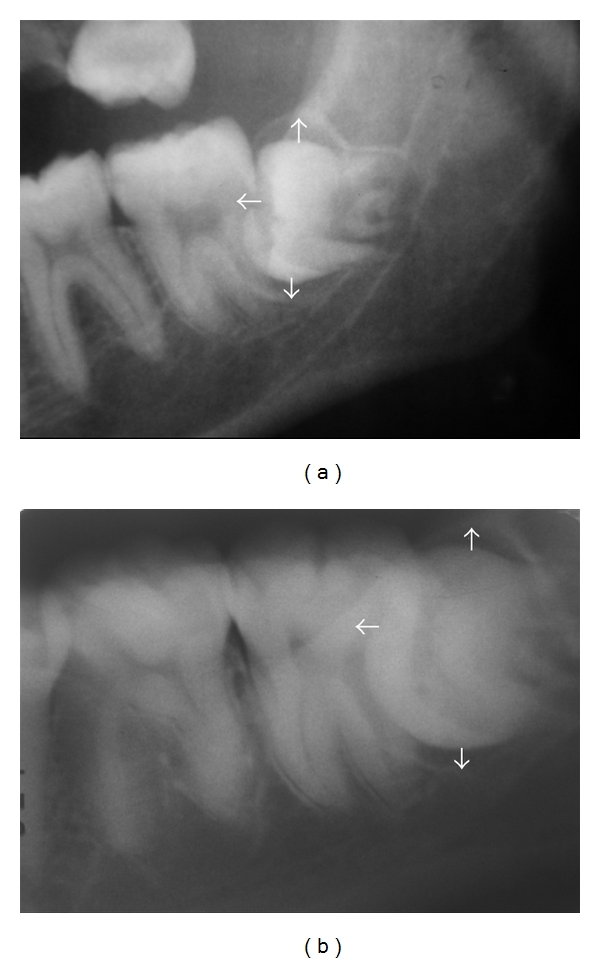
HPF in impacted mandibular third molar; 19-year-old female. (a) Panoramic radiograph. (b) Periapical radiograph of the same case.
